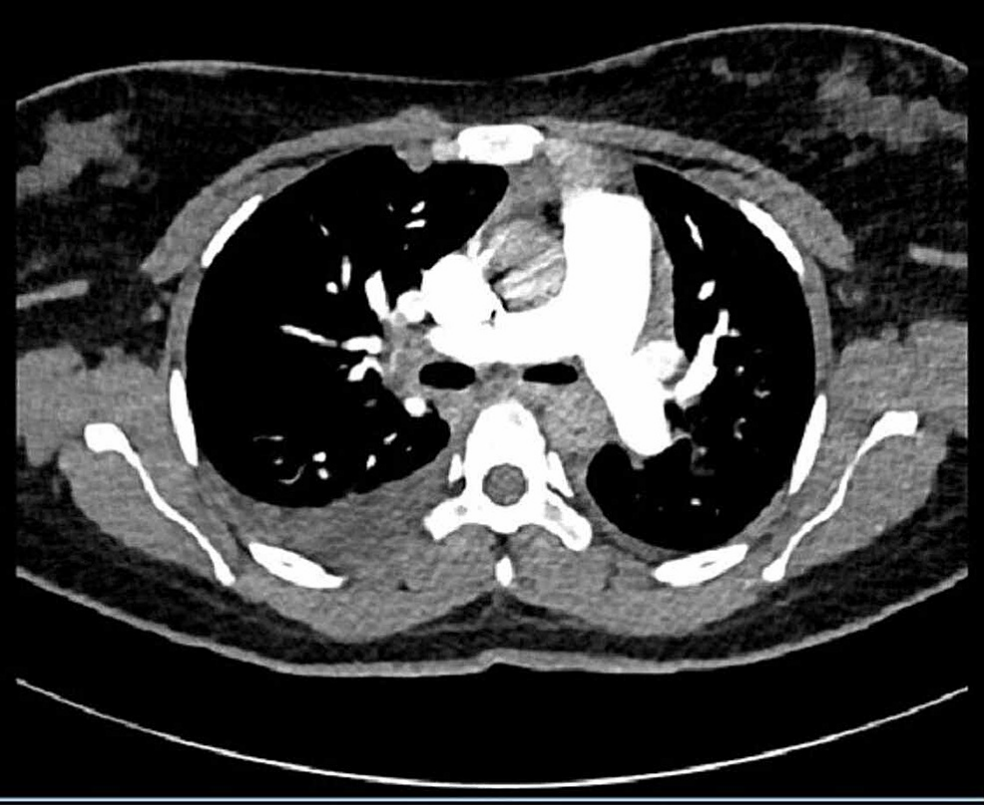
CT pulmonary angiogram showing no evidence of pulmonary embolism.
